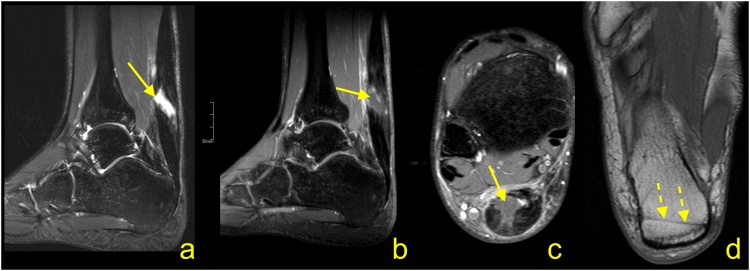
Status after Achilles tendon reconstruction 14 months ago. Patient presenting with pain in the midportion of the Achilles tendon. The skin over this area is somewhat changed, and reddening is visible. Clinical suspicion of infection. MRI with contrast was performed (a–d). At the level of the sutures, there is a visible substance defect in the tendon, which is oriented spirally (straight arrow) and filled with fluid (a). Only linear contrast enhancement is seen as no evidence of an abscess (b and c). Reinforcement sutures passing through the osseous tunnel in the calcaneus is seen on T1-weighted imaging (d - dashed arrow). a – T2-weighted with fat suppression, b and c- T1-weighted with fat suppression and iv contrast, d- T1- weighted.
